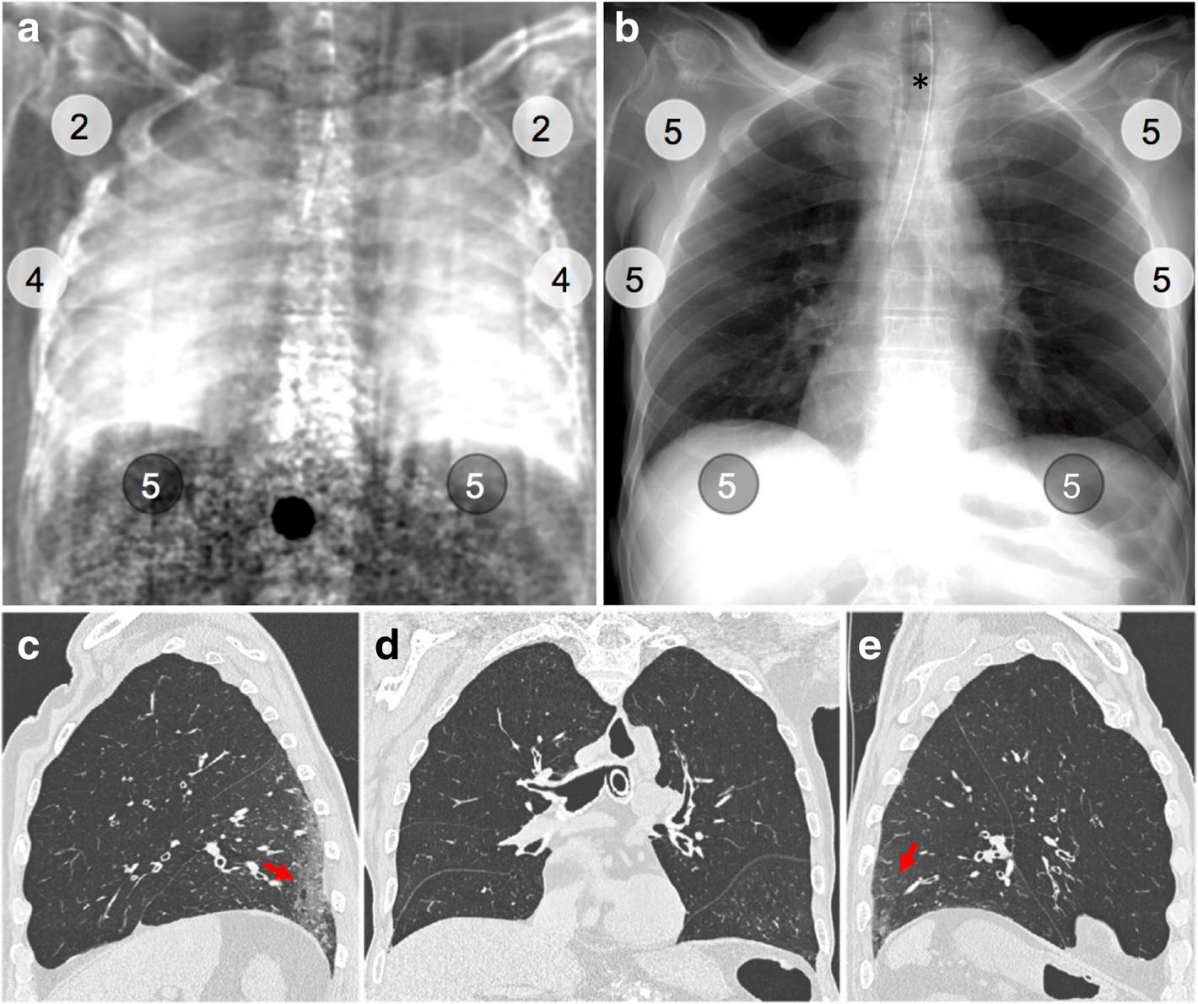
Dark-field chest radiograph, conventional x-ray, and CT of human body 4. Median visual grading (encircled numbers) of dark-field chest radiograph (a) by three independent readers shows increasing dark-field signal from the apex to the base of the lung. Median visual grading of transmission in conventional x-ray (b) shows no difference between upper, middle, and lower zones. Sagittal (c, e) and coronal (d) CT images show regular ventilation of emphysematous lung parenchyma except from some minor subpleural dystelectasis (red arrows) in posterior lower lobes. Asterisk marks the location of the endotracheal tube, which was repositioned between x-ray dark-field/conventional x-ray imaging and CT
